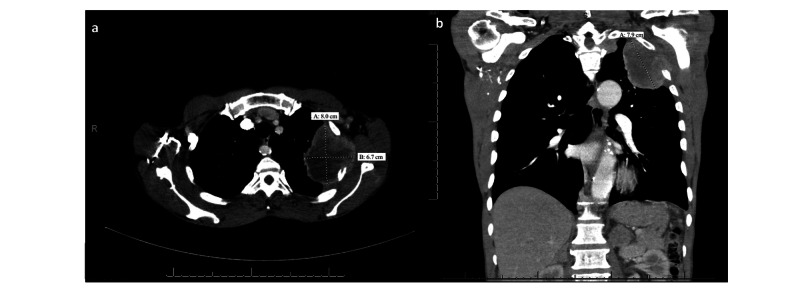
Axial and coronal CT images of the chest showing heterogeneous left apical mass Axial (a) and coronal (b) CT images of the chest demonstrating an 8.0 x 6.7 x 7.9 cm heterogeneous left apical mass with central necrosis and invasion into the left extra pleural space and chest wall with partial destruction of the second and third ribs.
